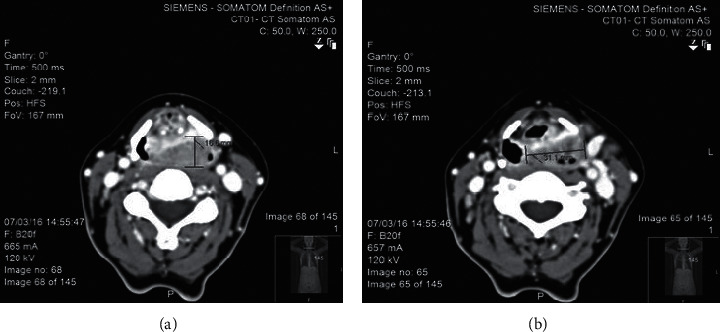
Prechemotherapy and radiotherapy CT scans. (a) and (b) show a left parpharyngeal mass measuring 31.1 mm in width and 16.6 mm in height compressing the airway from the ipsilateral side.
